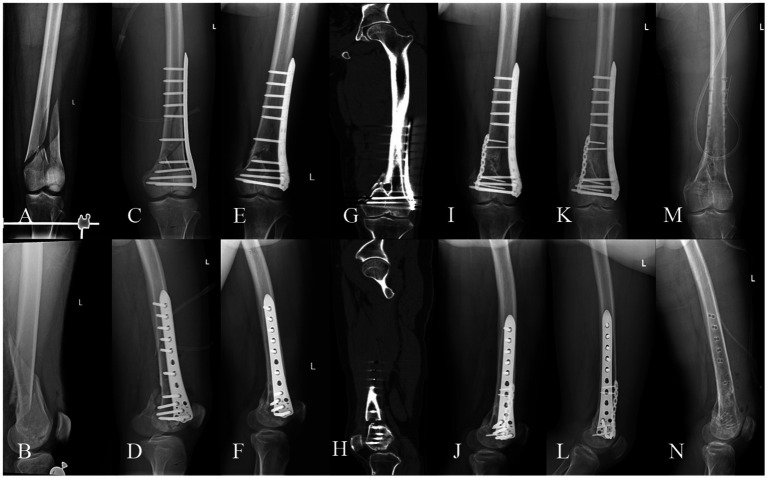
A 37-year-old male, BMI: 25.98 kg/m^2^, 2-year smoking history, no metabolic diseases. (A,B) Left distal femoral comminuted fracture sustained in a car accident on January 19, 2022. (C,D) Open reduction and lateral locking plate fixation performed on January 29, 2022. (E,F) Delayed union observed during follow-up on December 23, 2022. (G,H) Nonunion with anterior-medial cortical defect confirmed by CT on June 6, 2023. (I,J) Revision surgery on July 21, 2023, retaining the lateral locking plate and augmenting with an anterior-medial auxiliary plate, PRP-augmented structural iliac bone grafting, and bioactive core construction. (K,L) Radiographic union confirmed at 6.4 months postoperatively (February 2, 2024), with a lower extremity functional score of 60 and a VAS pain score of 0. (M,N) Implant removal performed on March 19, 2024.
